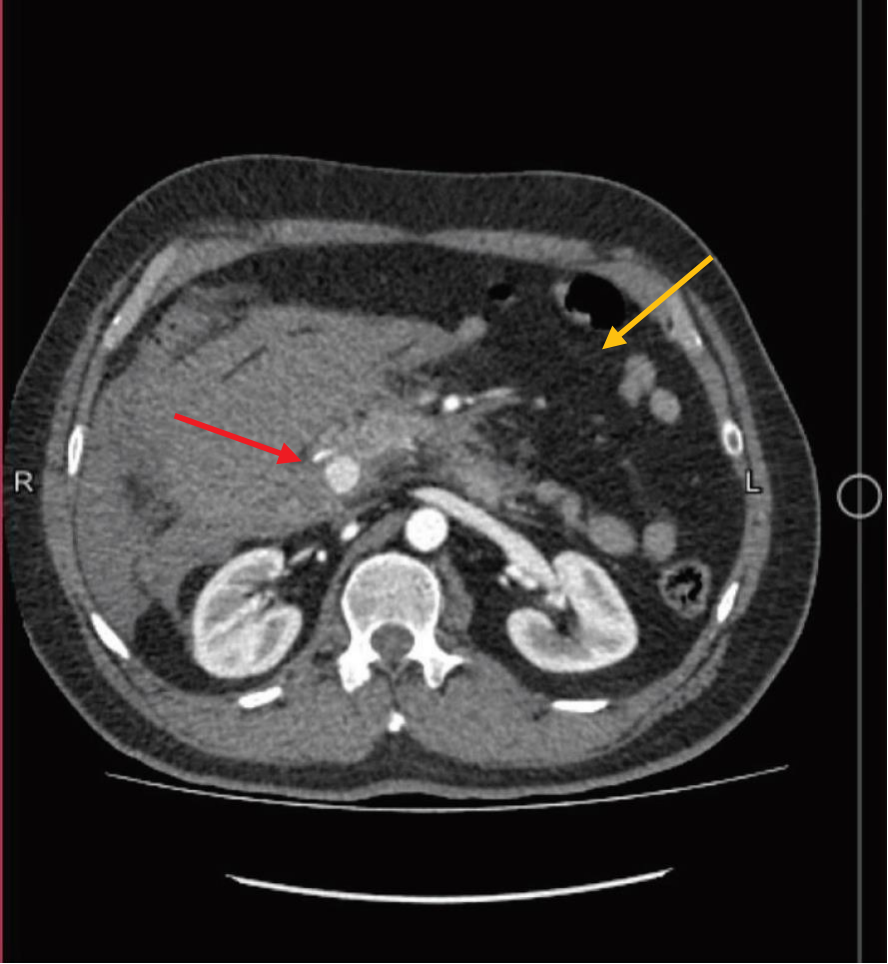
IV contrast‐enhanced CT angiography showing enlarged iso‐to‐hyperdense lesions with significant inflammatory changes around the duodenum, peripancreatic, gastroduodenal, proximal pararenal, and right lateroconal in favor of a large hematoma with a reactive diameter increase of the duodenum and ascending colon (yellow arrow). It also shows a saccular outpouching image consisting of contrast with an irregular wall in the peripancreatic zone in superior and inferior pancreaticoduodenal anastomosis in favor of visceral aneurysm, probably with the origin of the inferior pancreaticoduodenal artery (red arrow). CT, computed tomography.
